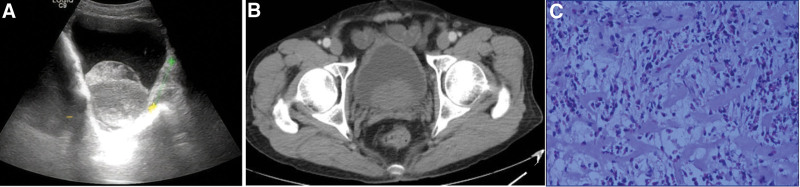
Imaging examination and pathological results of eosinophilic cystitis (A) B-ultrasound image; (B) CT image; (C) postoperative pathology (HE, ×400).
